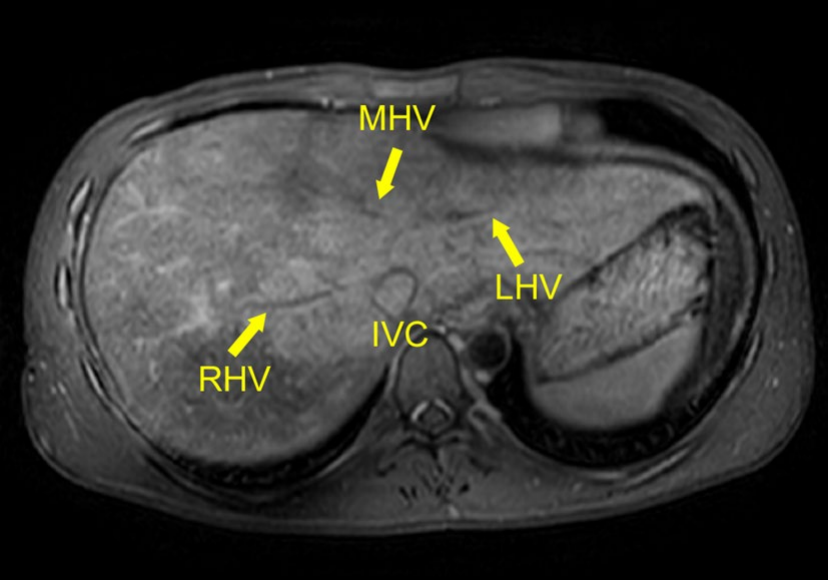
Preoperative abdominal magnetic resonance imaging (MRI) showing obstructed and scarred main hepatic veins. Abbreviations: IVC, inferior vena cava; LHV, left hepatic vein; MHV, middle hepatic vein; RHV, right hepatic vein
